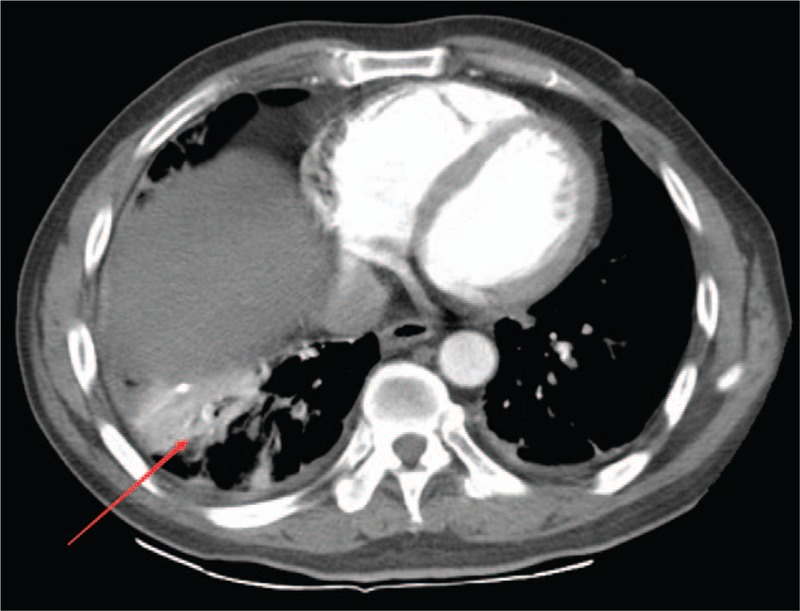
CTPA revealed right lower pulmonary infarctions (arrow) and a small amount of pleural effusions on both sides. CTPA = computed tomography pulmonary angiography.
